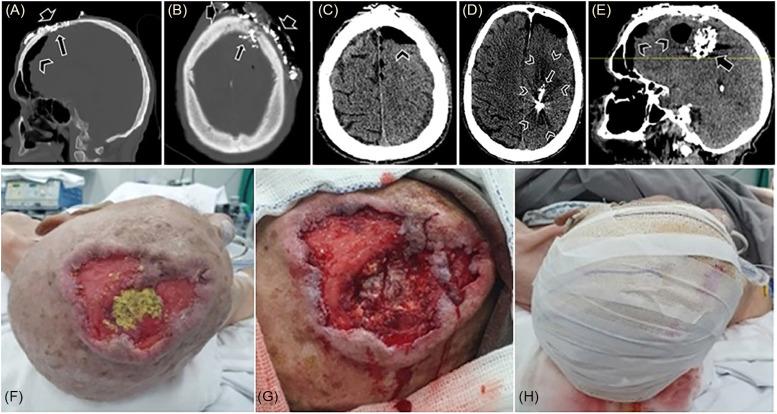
First CT scan: bone window, sagittal (A) and axial (B) planes – osteolytic lesion in the frontal bone (long arrow) establishing communication between the meningeal membranes and the external environment through an extensive ulcerated lesion (short arrow) in the skin and subcutaneous tissue. Pneumocephalus (arrowhead); (C) parenchymal window, pneumocephalus (arrowhead). Second CT scan: parenchymal window: (D) axial plane – hypodense lesions in the left frontal and parietal lobes, suggesting organized cerebritis/abscess with possible areas of necrosis (arrowhead); multiple metallic-density particles intermingling these tissues, representing iodoform (long arrow); (E) sagittal plane – iodoform particles (arrow) and pneumocephalus (arrowhead); (F) extensive irregular wound measuring 7.0 cm × 8.0 cm, with raised and erythematous edges; presence of gauze with Iodoform in the region of greatest excavation, corresponding to the osteolytic lesion; (G) post-debridement local appearance showing exposed meningeal membranes; (H) local occlusive dressing with Rayon, Rifocin, and Dersani gauze.
